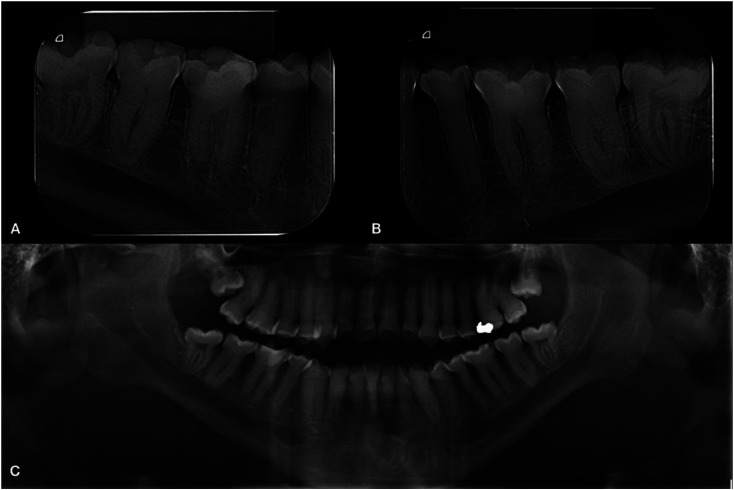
Radiographic examination. A, B: Periapical radiographic showing the teeth with absent pulp chamber and obliterate root canals (third molar exception) and bilateral mandibular canal increase, right and left side. C: Panoramic radiographic showing these findings.
